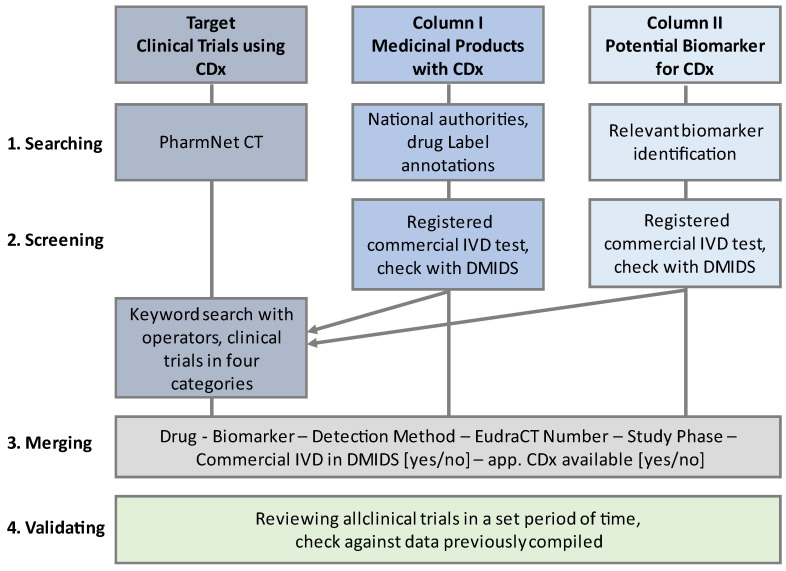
Concept of the search strategy for identifying companion diagnostics applied in clinical trials in the EU using the PharmNet CT database. The search in PharmNet CT was based on collected data of medicinal products in personalized medicine and associated biomarkers which are potentially eligible for in vitro diagnostic testing. Information on commercially available in vitro diagnostic medical devices and the applied detection method were gathered from DMIDS (German Medical Devices Information and Database System [11]). An advanced keyword search in PharmNet CT was conducted. The results from this search were documented based on the EudraCT number of the trial.
